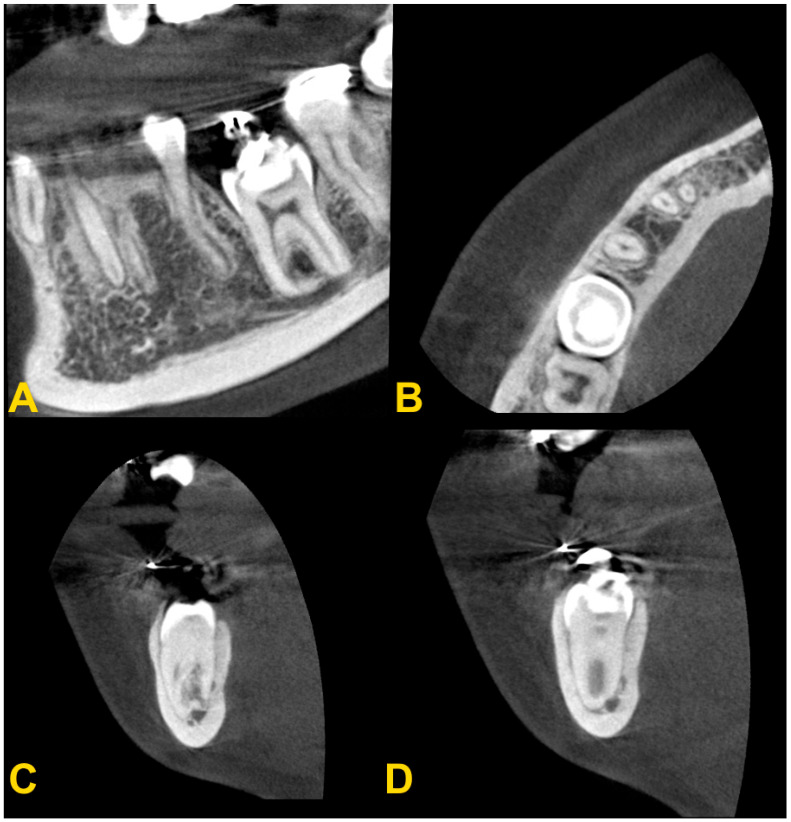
Cone-beam computed tomography examination of tooth no. 36 with infraocclusion: (A) panoramic reconstruction, (B) axial view, (C) transectal view through the mesial root, and (D) transectal view through the distal root (the same patient as in Figure 6D).
